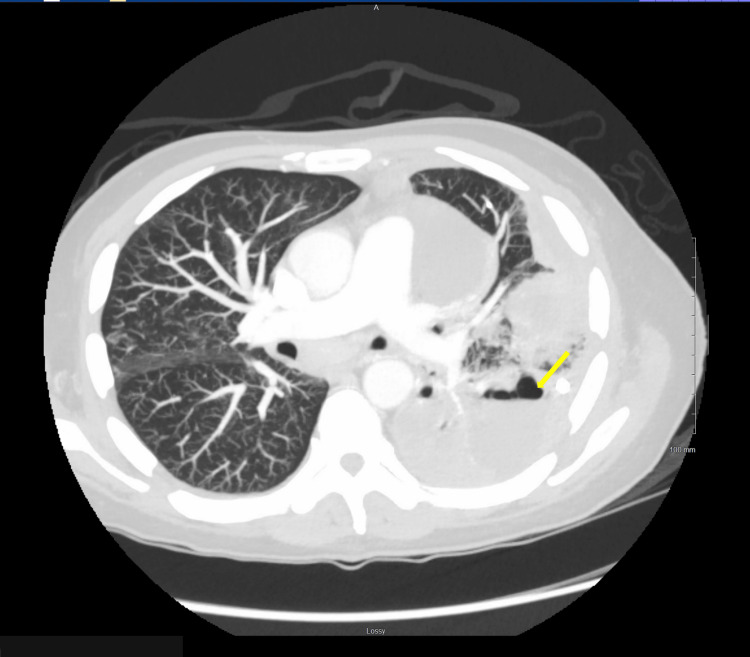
Computed tomography scan of the chest demonstrating the opacification and necrotization of the left lower lobe cavitary lesion
